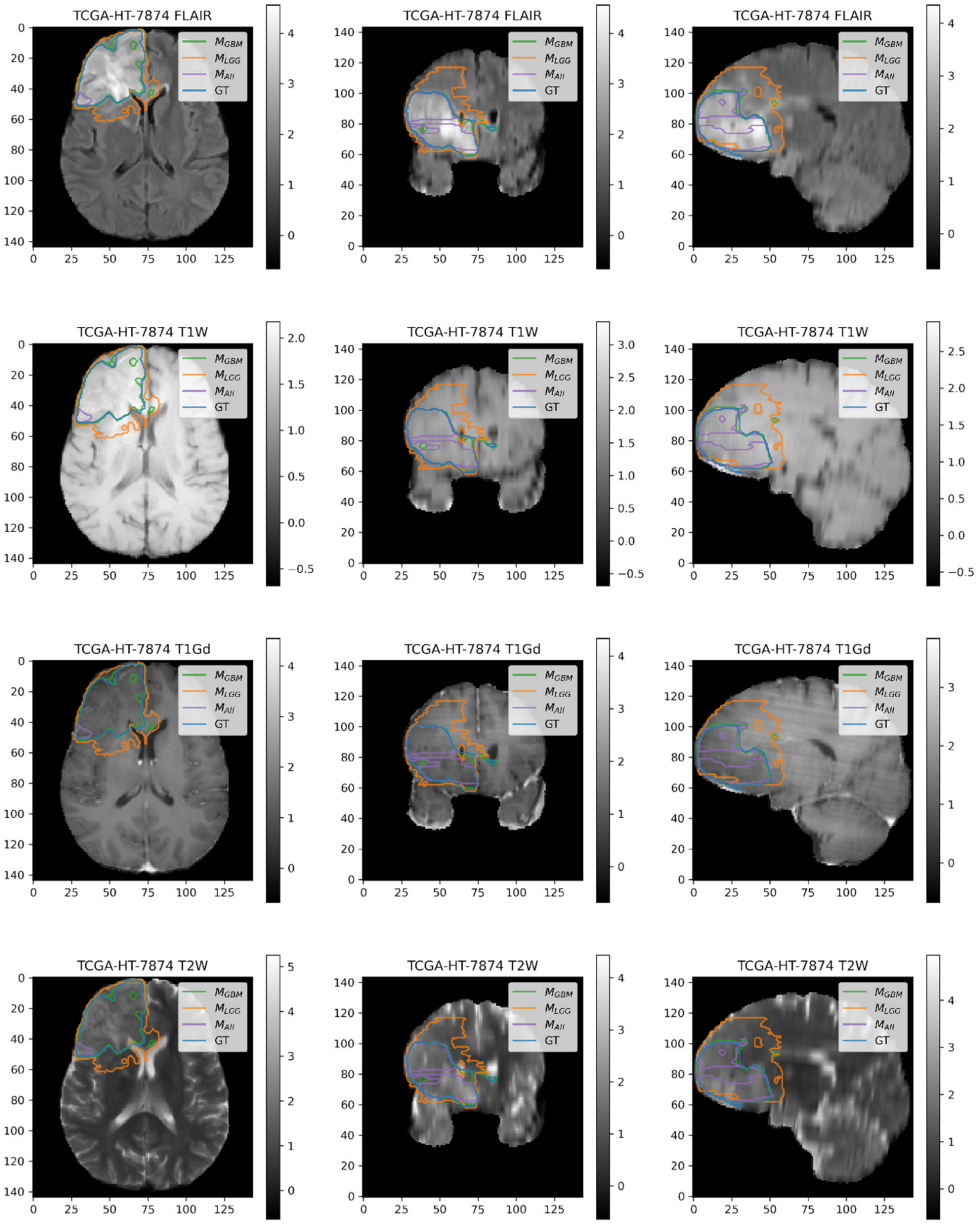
Patient TCGA-HT-7874 belongs to *D*_*LGG*_ and has a tumor in the right frontal lobe. We selected this patient as it has consistently worse performance for metrics (Sens, B.Acc, Dice, Jac.C) across all models. Segmentation plot indicates *M*_*All*_ and *M*_*GBM*_ under-segment in this case, whereas *M*_*LGG*_ over-segments. *M*_*ALL*_ appears to be missing a central part of the tumor, as seen in the coronal and sagittal image planes. *M*_*LGG*_ appears to extend well beyond the region of FLAIR enhancement to over-segment the tumor. This LGG was significantly larger than most LGGs, and that may contribute to the difficulty of segmentation.
